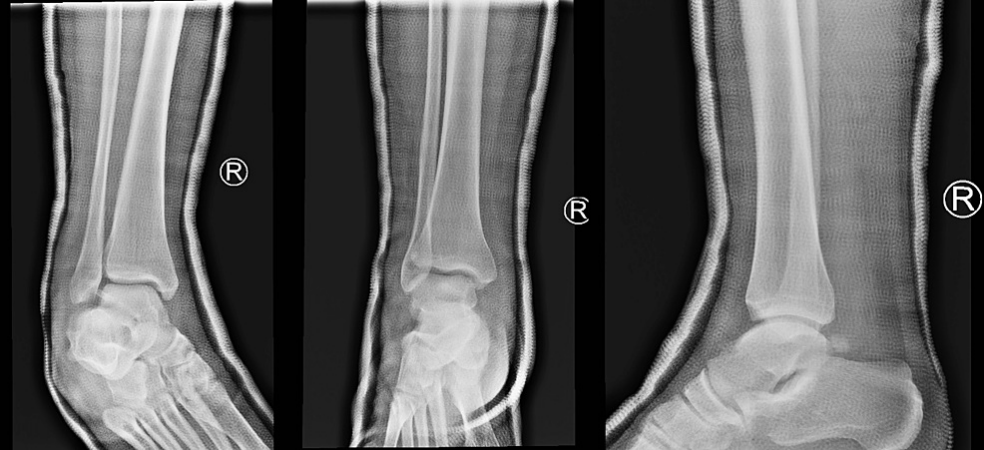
Plain X-rays at two-week follow-up. The back slab was changed for below-knee circular cast.
